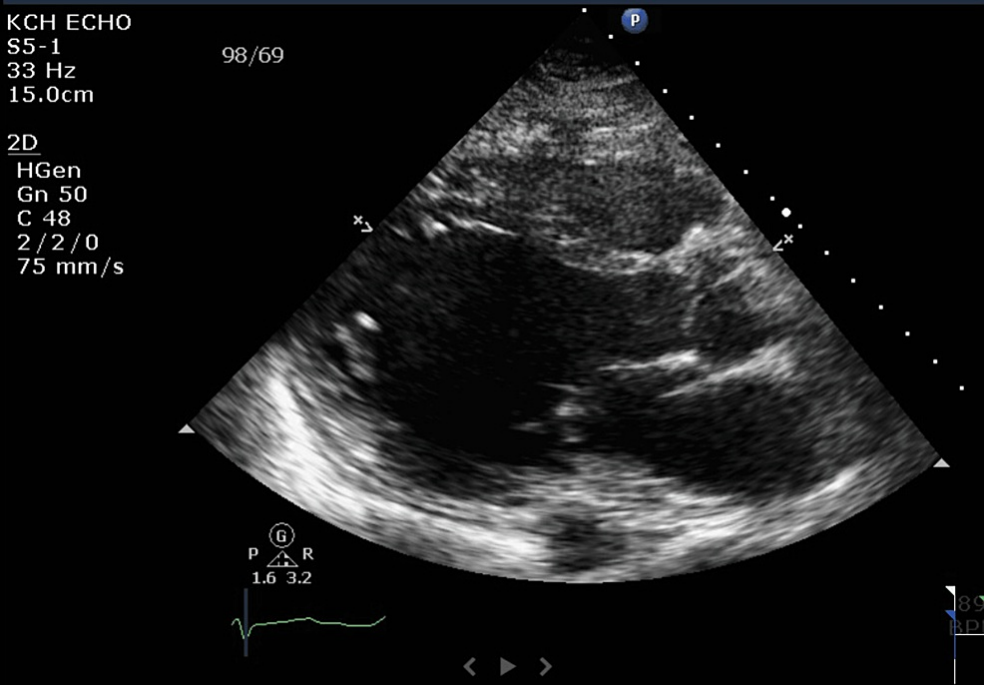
TTE on 12/6/2022 showing the end-diastole phase with LVEF 5% TTE: transthoracic echocardiography; LVEF: left ventricular ejection fraction
